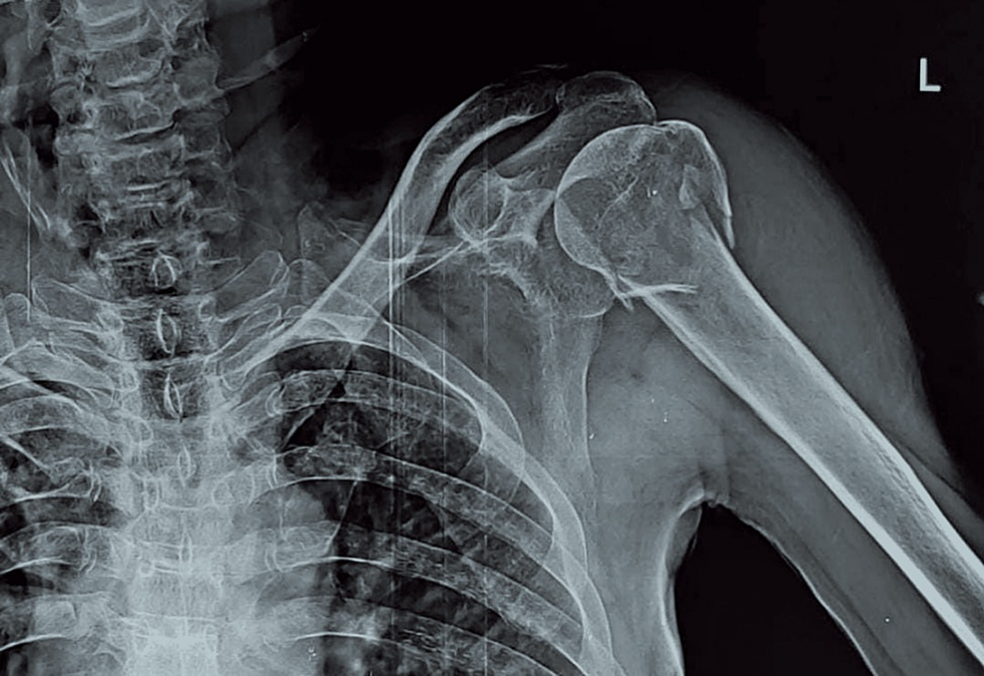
Plain radiograph antero-posterior view showing three-part proximal humerus fracture
